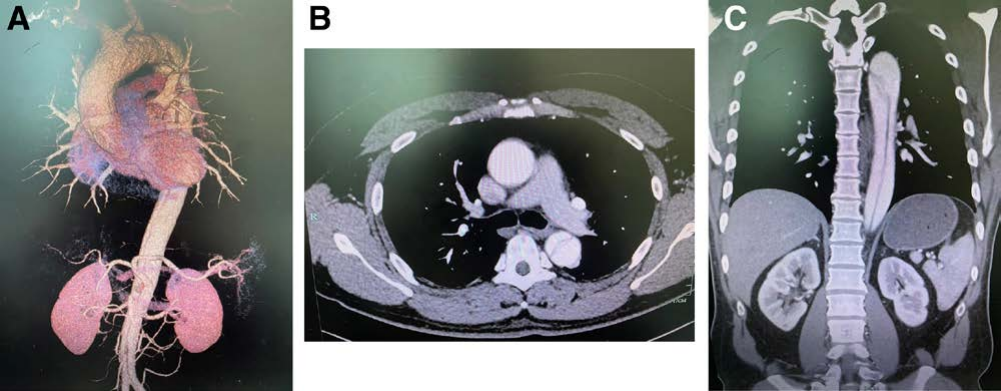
The CTA shows a type B aortic dissection with different anatomical features. (A) Three-dimensional image, (B) transverse section, and (C) coronal section.
